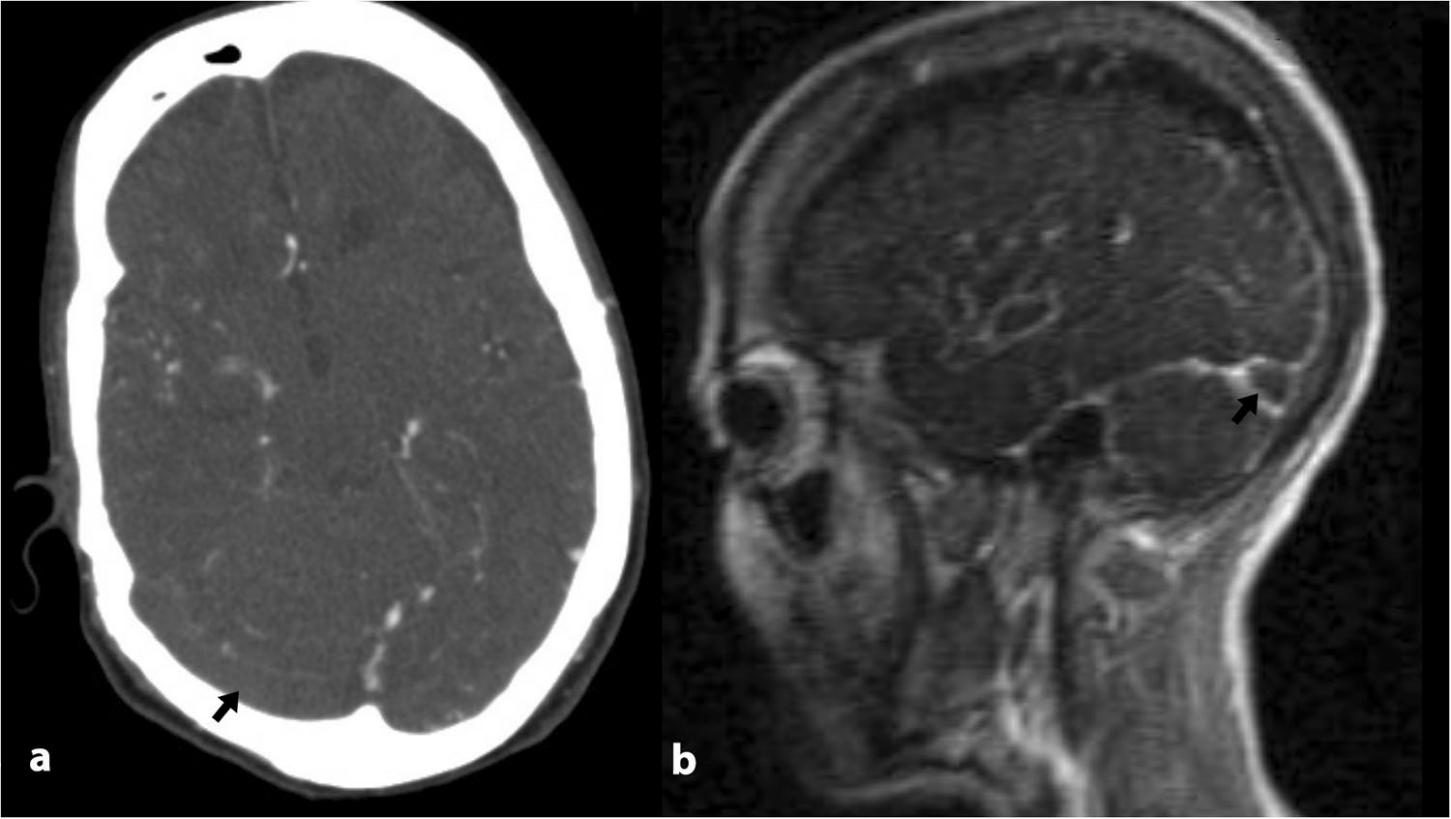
(a) Axial brain CT angiography shows filling defect in the right transverse sinus (black arrow). (b) Sagittal contrast enhanced brain MR venography shows filling defect in the right transverse sinus (white arrow).
